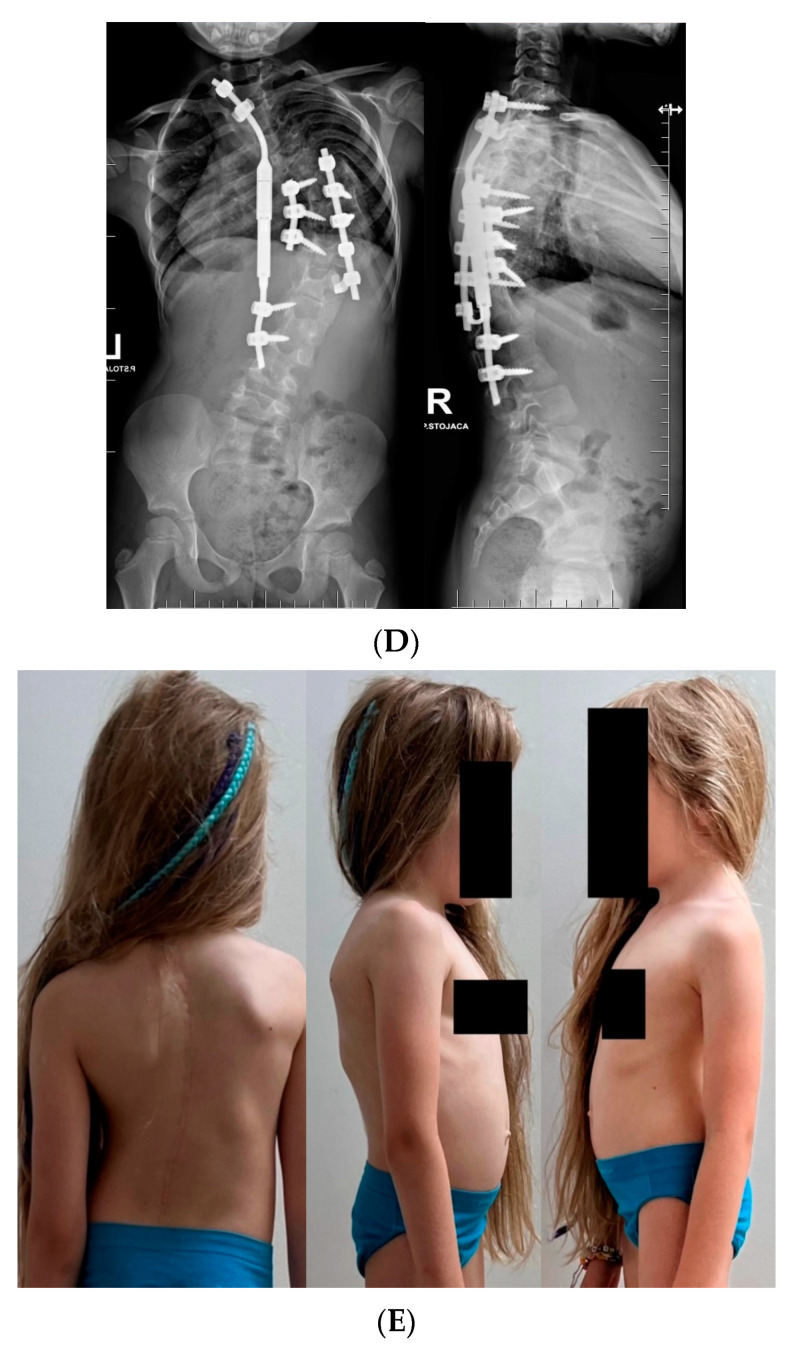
Five-year-old girl with severe congenital scoliosis (multilevel hemivertebrae and blocked vertebrae) reaching 120° (A,B). A preoperative HGT was used for 3 months (C), as well as two levels of P-VCR with short fusion and a single-MCGR construct for distraction left side. X-rays (D) and clinical pictures before the treatment course and after the 2-year follow-up period (E).
